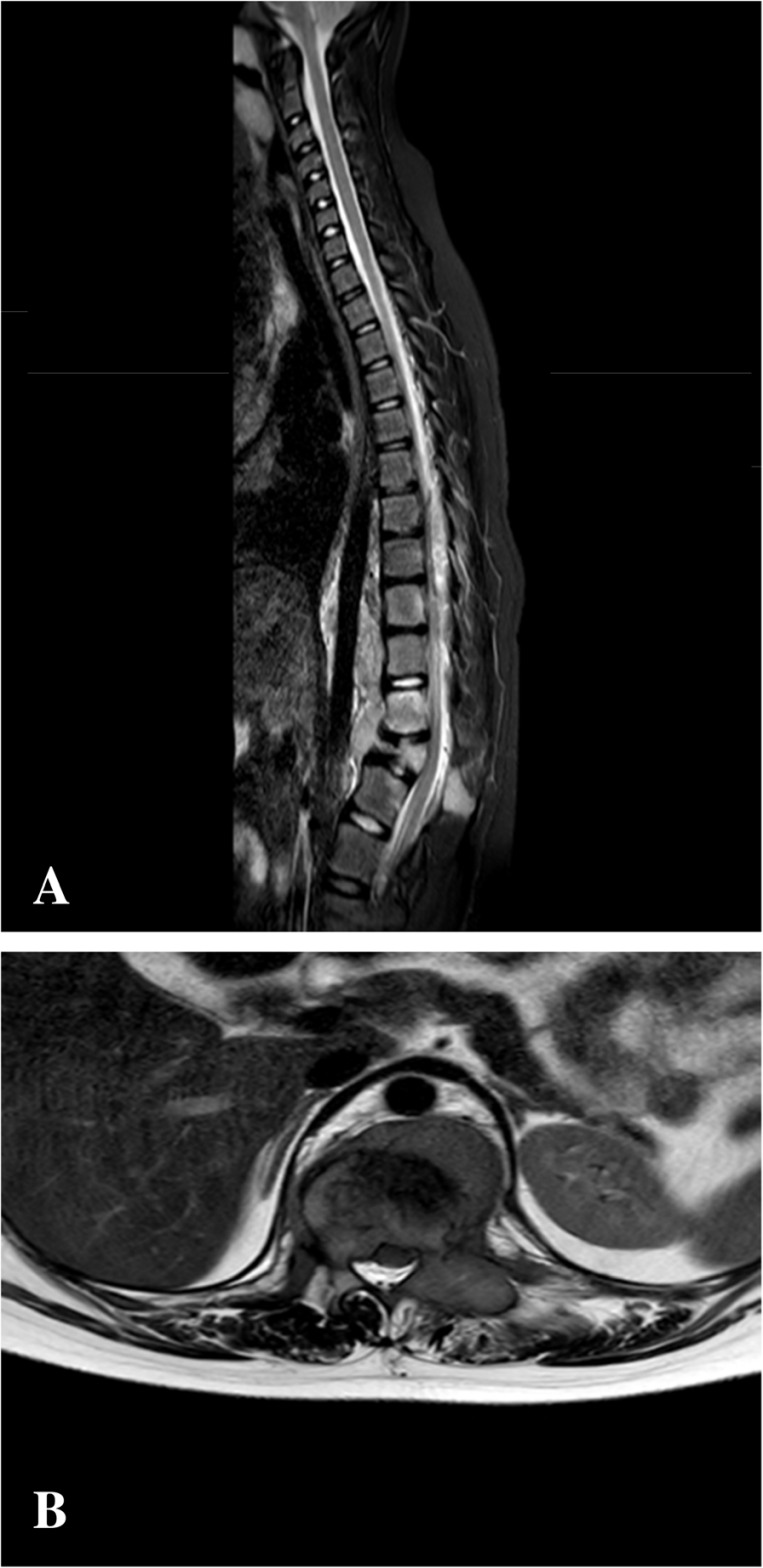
Initial magnetic resonance imaging of the spine. Magnetic resonance imaging of the spine, fat-saturated T2-weighted sagittal plan (A) and T2-weighted axial plan (B), revealing an epidural mass causing collapse of twelfth thoracic vertebra and compression and displacement of the spinal cord from Th12 to L1
